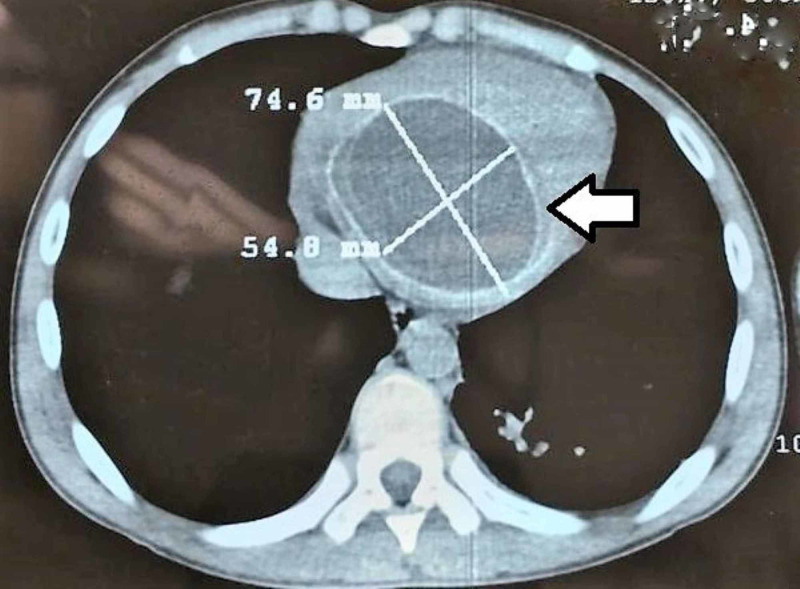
CT scan of the chest showing the intramyocardial cyst (axial view).
